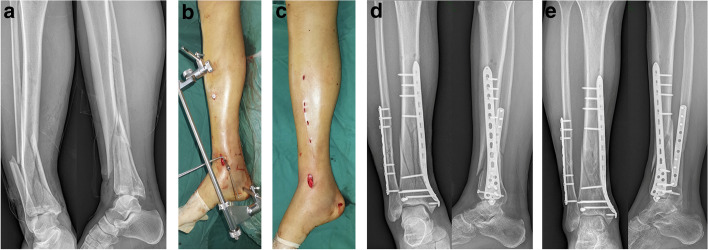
A–E An AO distractor was used to achieve satisfactory reduction of a distal tibia fracture. A Anteroposterior and lateral radiographs of a male who sustained an AO 43-C1.3 type distal tibia fracture. B An AO distractor was used to achieve satisfactory reduction. C Final view of operated limb after completion of MIPO. D Postoperative anteroposterior and lateral radiographs. E Anteroposterior and lateral radiographs 6 months postoperative showing a healed distal tibia
